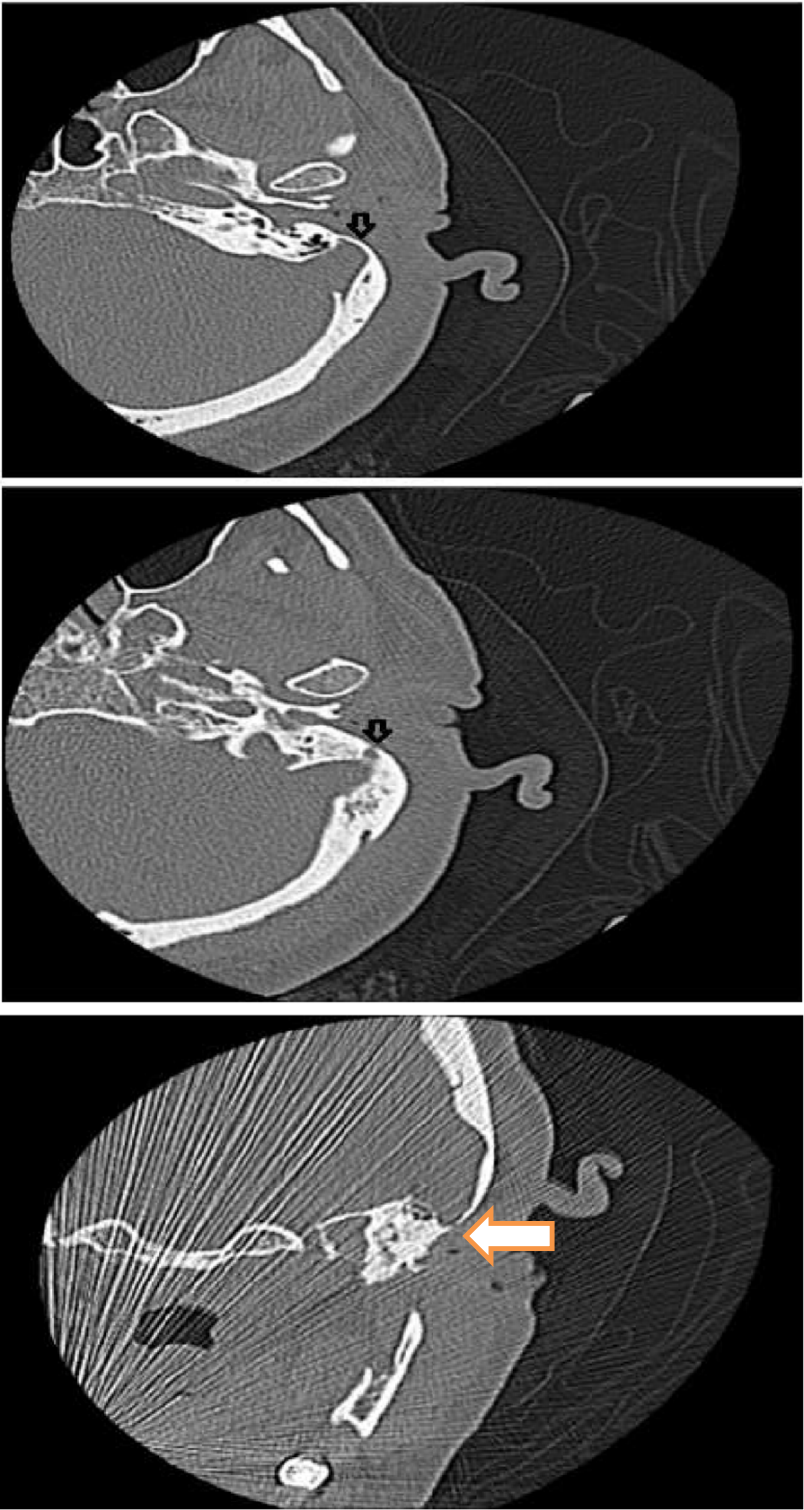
A postoperative high-resolution computed tomography (HRCT) of the temporal bone shows that the vertical segment of the left sigmoid sinus (arrow) is abnormally forward- displaced
